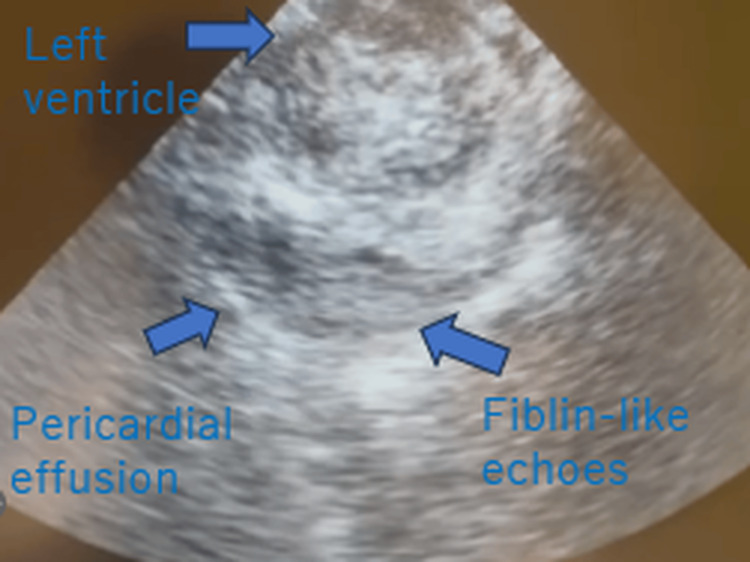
Transthoracic echocardiography showing pericardial effusion with fibrinous strands. Transthoracic echocardiography demonstrating pericardial effusion surrounding the left ventricle with echogenic fibrinous strands, consistent with organized hemopericardium associated with ventricular free wall rupture. Arrows indicate fibrinous echoes within the pericardial effusion adjacent to the left ventricular free wall.
